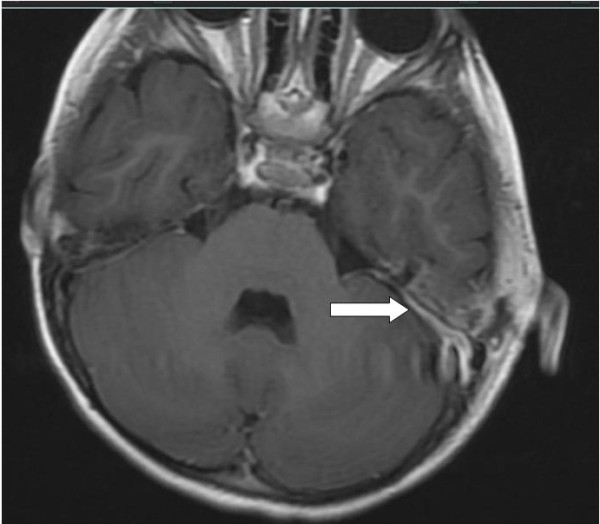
MRI horizontal section T1 + Gadolinium. Arrow shows local thickening and enhancement of the dura.
